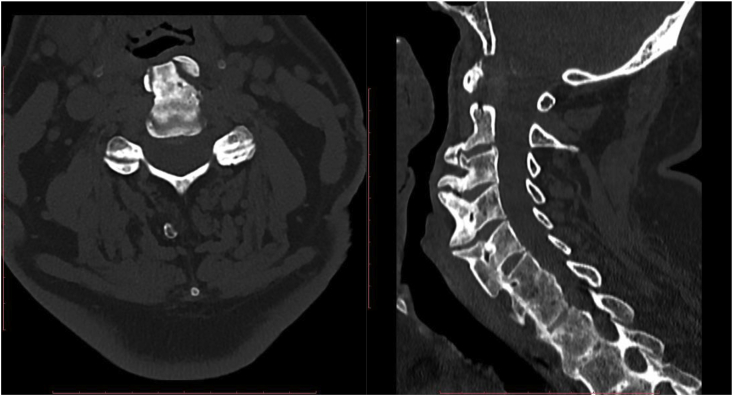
Sagittal computed tomography reconstruction of the cervical spine of a 73-year-old man with diffuse idiopathic skeletal hyperostosis and moderate dysphagia demonstrating extensive ossifications ventral of C2–C7.
